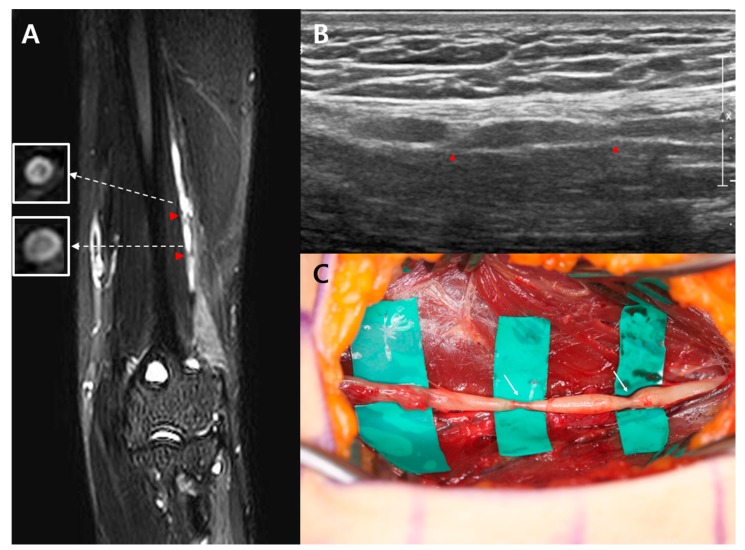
Case 2: (A) High-resolution magnetic resonance neurography reveals two focal constrictions (red arrowhead) of the left radial nerve 8.0 and 6.9 cm proximal to the lateral epicondyle, respectively. A bull’s eye sign (in white square) is observed juxta-proximal to each constriction site of the left radial nerve; (B) Longitudinal ultrasound reveals two constrictions (red arrowheads) of the left radial nerve; (C) Intraoperative findings confirm two constrictions (write arrow) of the left radial nerve.
